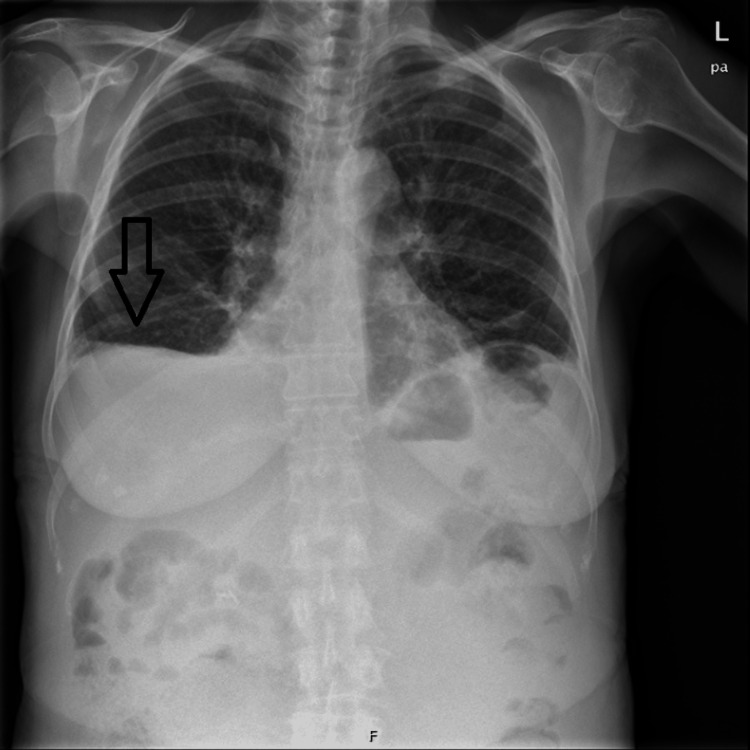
Chest radiograph at admission showing pleural effusion
